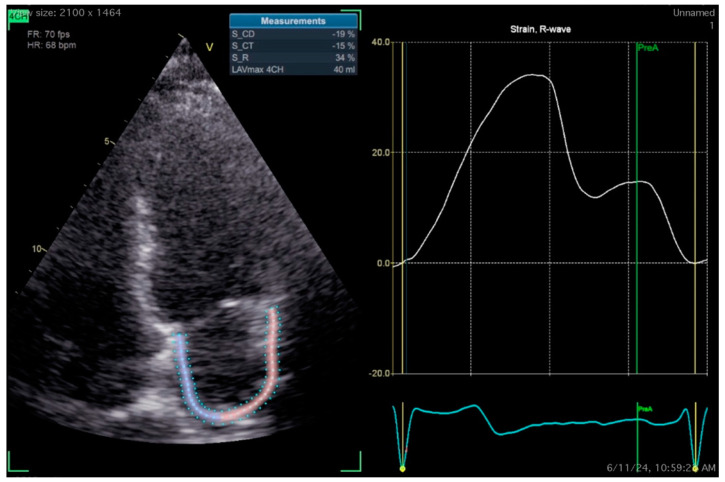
Abnormal left atrial strain parameters by 2D-STE in a 44-year-old male patient with paroxysmal atrial fibrillation in sinus rhythm. Transthoracic echocardiogram was performed 24 h before pulmonary vein isolation with pulsed-field ablation.
